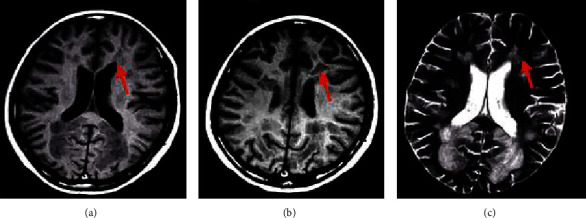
WML MRI image of a patient. (a), (b), (c) The flair manifestation, T1WI, and T2WI, respectively.
